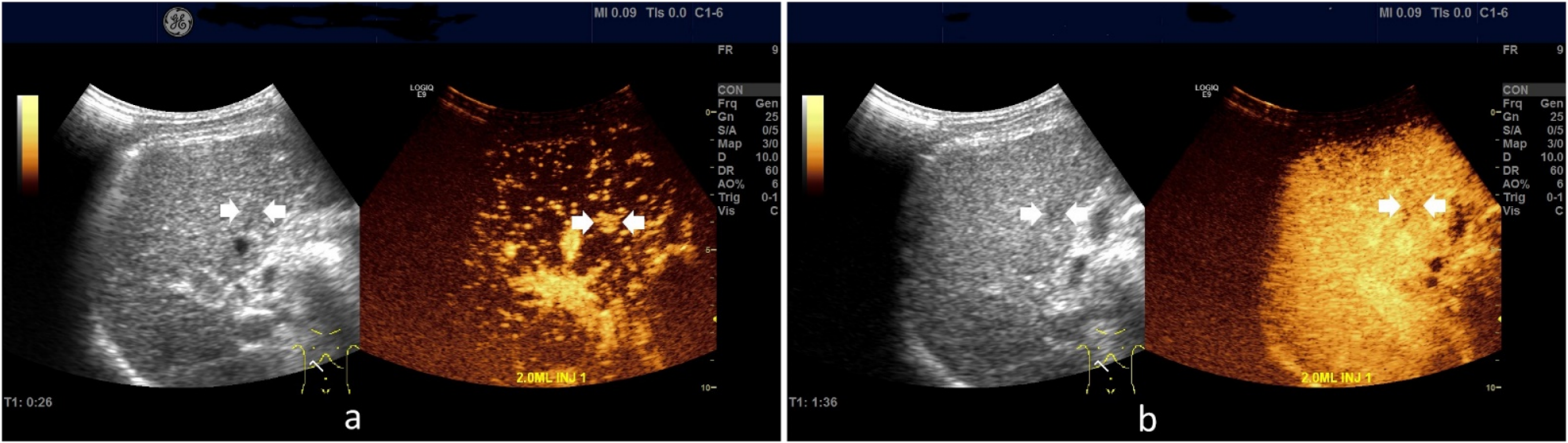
Images showed a nodule classified as LR-5 in a 62-year-old male with chronic hepatitis B virus-related cirrhosis and pathologically confirmed HCC. A 13 mm nodule located in the right lobe of the liver showed homogeneous hyperenhancement (arrow) in the arterial phase on contrast-enhanced ultrasound (a). A mild washout (arrow) was observed in the portal-venous phase (> 60 seconds; timer, 01:36) (b). The lesion was classified as LR-5 according to the CEUS LI-RADS.
